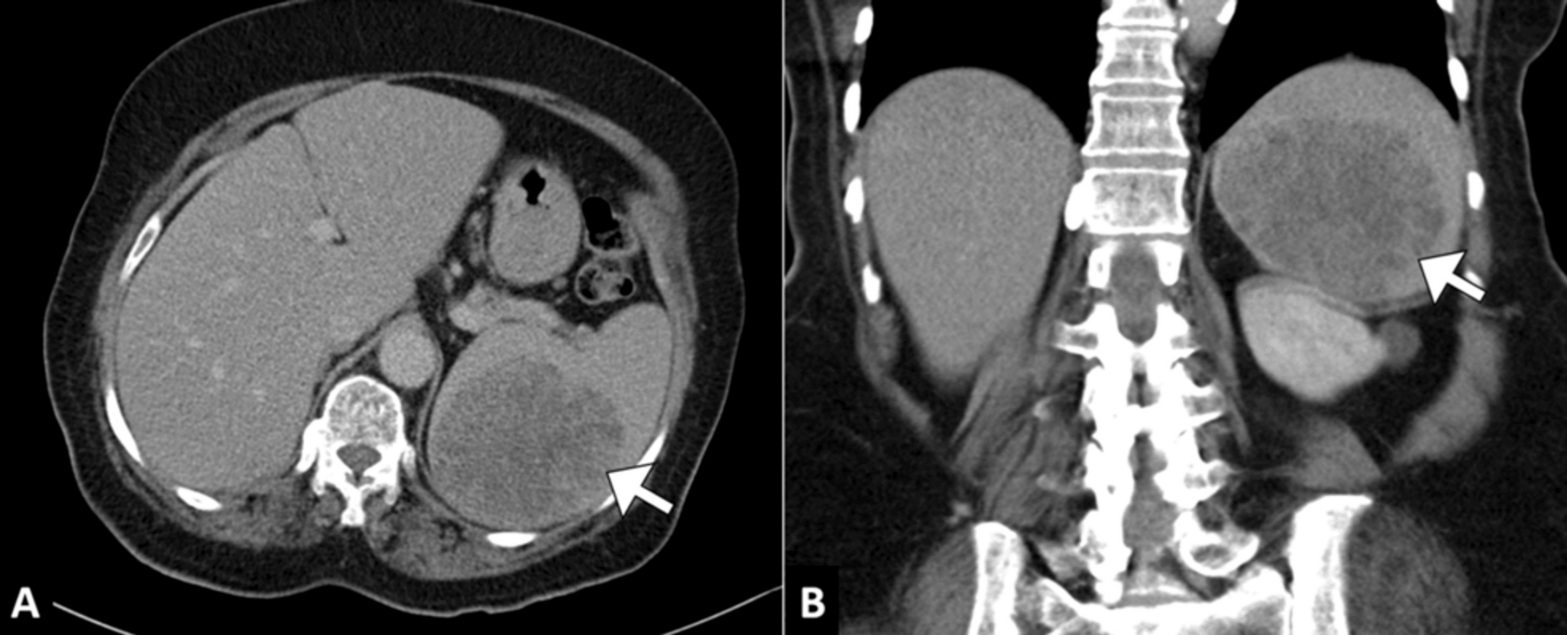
Isolated splenic metastasis on follow-up CT Axial (A) and coronal (B) contrast-enhanced CT images show a solitary, heterogeneous splenic lesion (arrow) with irregular margins and central necrosis, consistent with metastatic disease.
